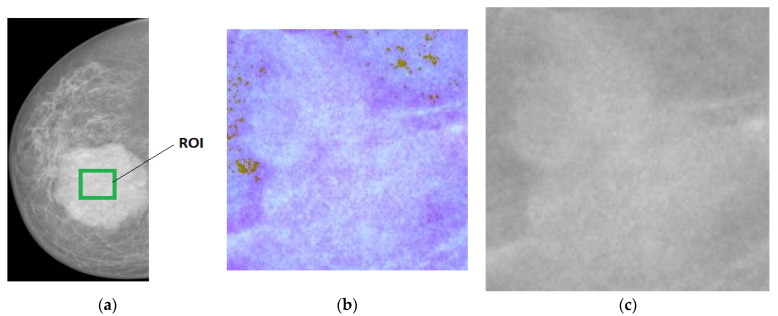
Example of data preparation phase for generating the pseudo-colored image based on the original grayscale X-ray mammogram. (a) Region of interest (ROI); (b) Pseudo-Colored image; (c) Grayscale image.
